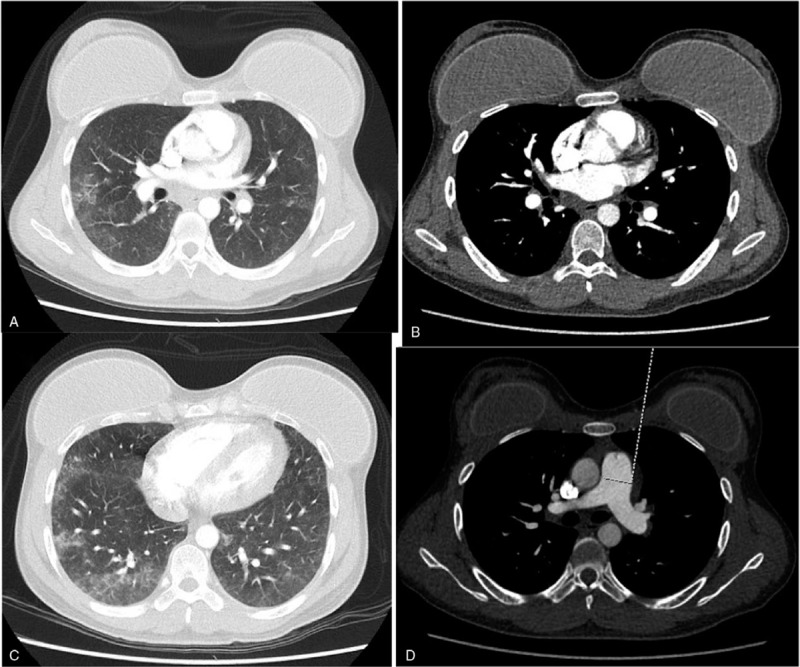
A and C. Thoracic CT angiography, lung window, with multiple infiltrates of alveolar occupation accompanied by ground glass patches, presence of breast prostheses. B and D. Mediastinal window showing pulmonary circulation without evidence of venous thromboembolism and aspect of the normal pulmonary artery. CT = computed tomography.
